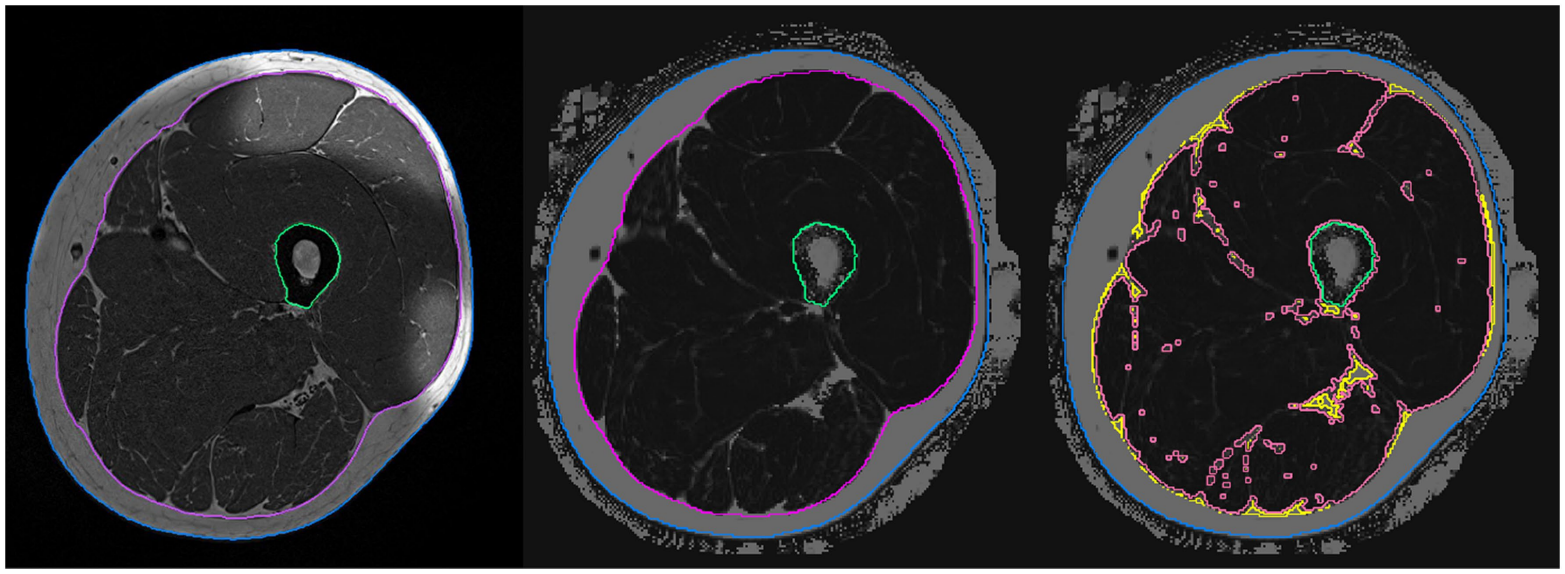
Segmentation and analysis of the magnetic resonance images. Left: T_1_-weighted image used for segmentation of the fascia (magenta), outline (blue), and femur (green). Center: Dixon image after registration of the analysis volumes. Right: Dixon image – separation into IMAT (yellow) and MT (light red) volumes.
